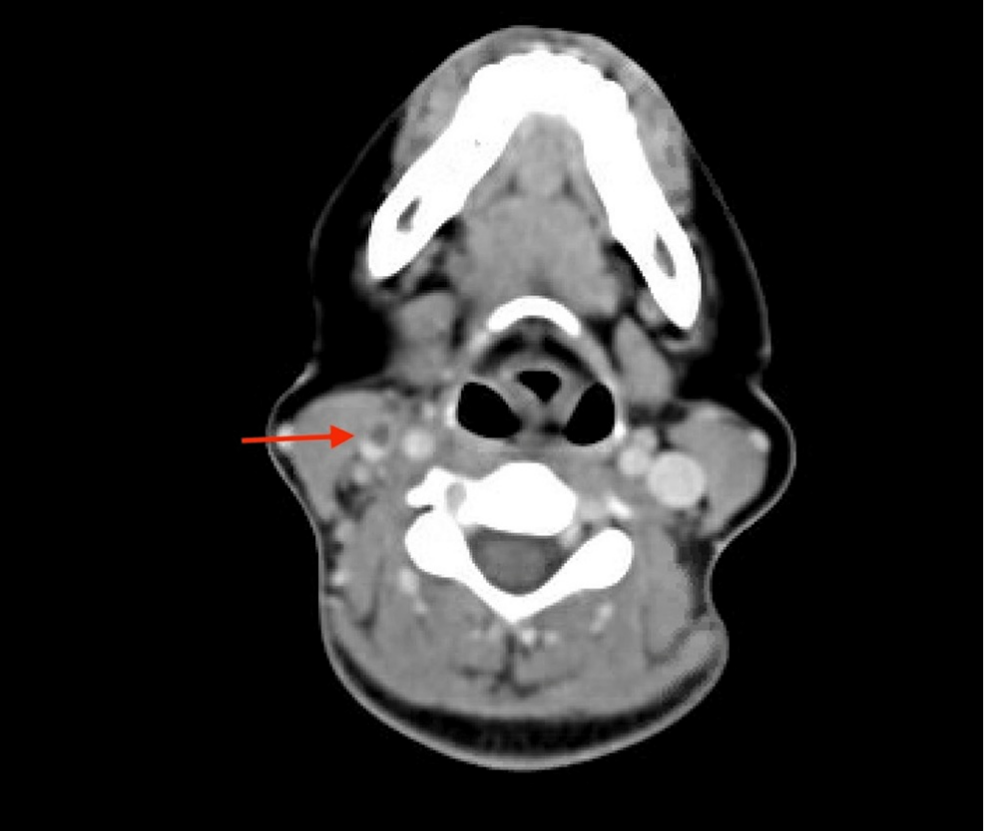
CT head (axial) Filling defect consistent with acute thrombophlebitis of the right internal jugular vein (red arrow). There is an asymmetric appearance of the right palatine tonsil pillar without evidence of a well-defined tonsillar abscess. Retropharyngeal edematous changes at the level of the oropharynx, and hypopharynx extending caudally to the supraglottic level
